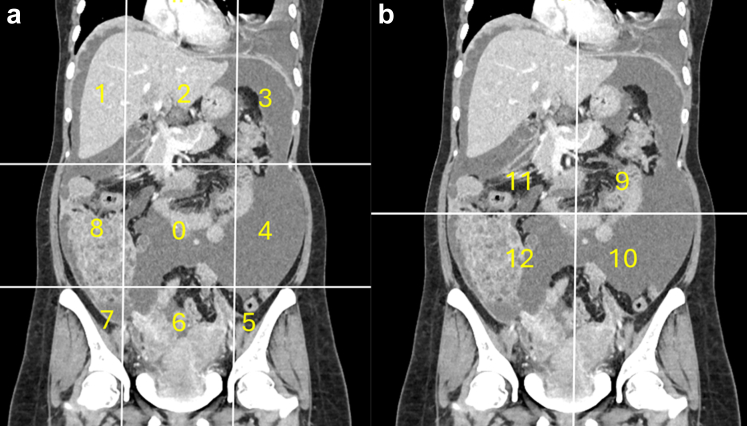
Calculation of radiological peritoneal cancer index (rPCI) adapted from Sugarbaker et al. (1). (a) Coronal CT showing regions 0–8. Vertical lines are drawn along the right and left midclavicular line and the horizontal lines along the costal margin and the iliac crests. (b) Coronal CT showing regions 9 to 12. The vertical and the horizontal lines are through the umbilicus divide the small bowel and mesentery into proximal and distal jejunal and ileal regions.
